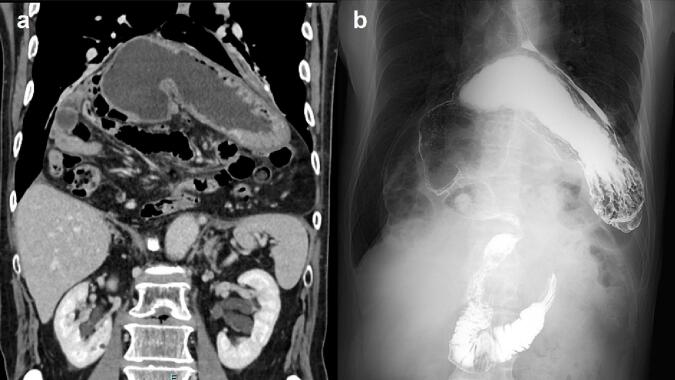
Coronal computed tomography showing (a) the intrathoracic stomach with organo-axial gastric volvulus and a large section of the small intestine. (b) Upper gastrointestinal series showing a volvulated stomach.
